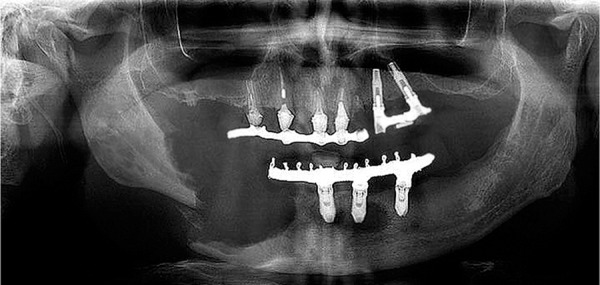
Panoramic radiography presenting a pathological fracture of the mandible on the right side.
